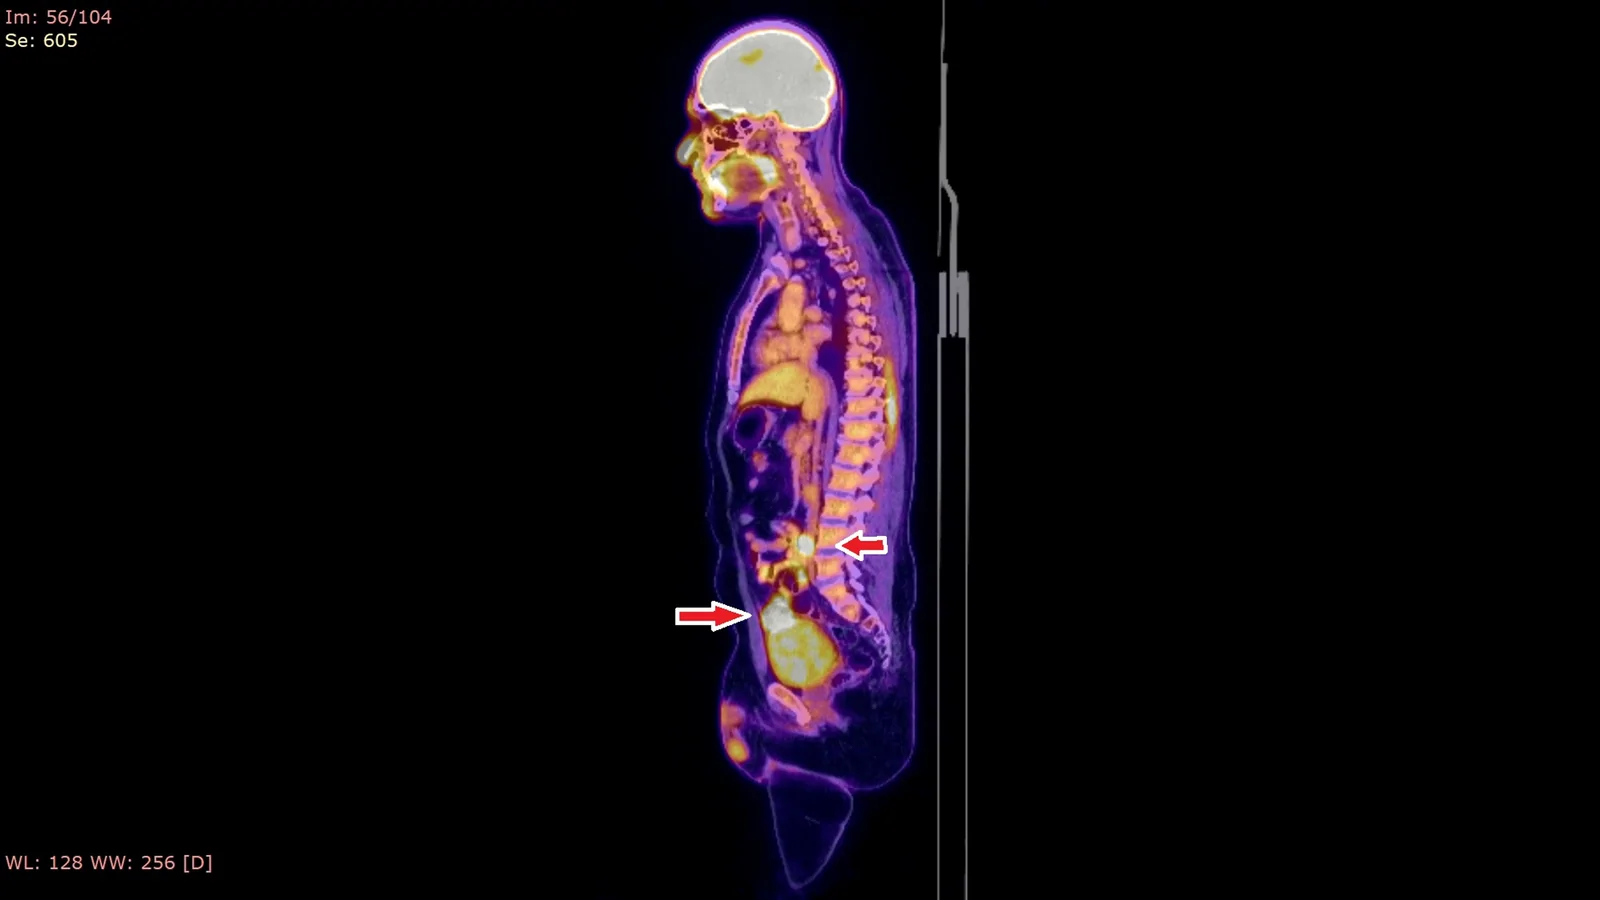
Fluorodeoxyglucose PET/CT image. The image shows fluorodeoxyglucose distribution with abnormal uptake at the bladder dome, with cranial extension of the lesion, as well as focal uptake in the common iliac lymph nodes (red arrows).
